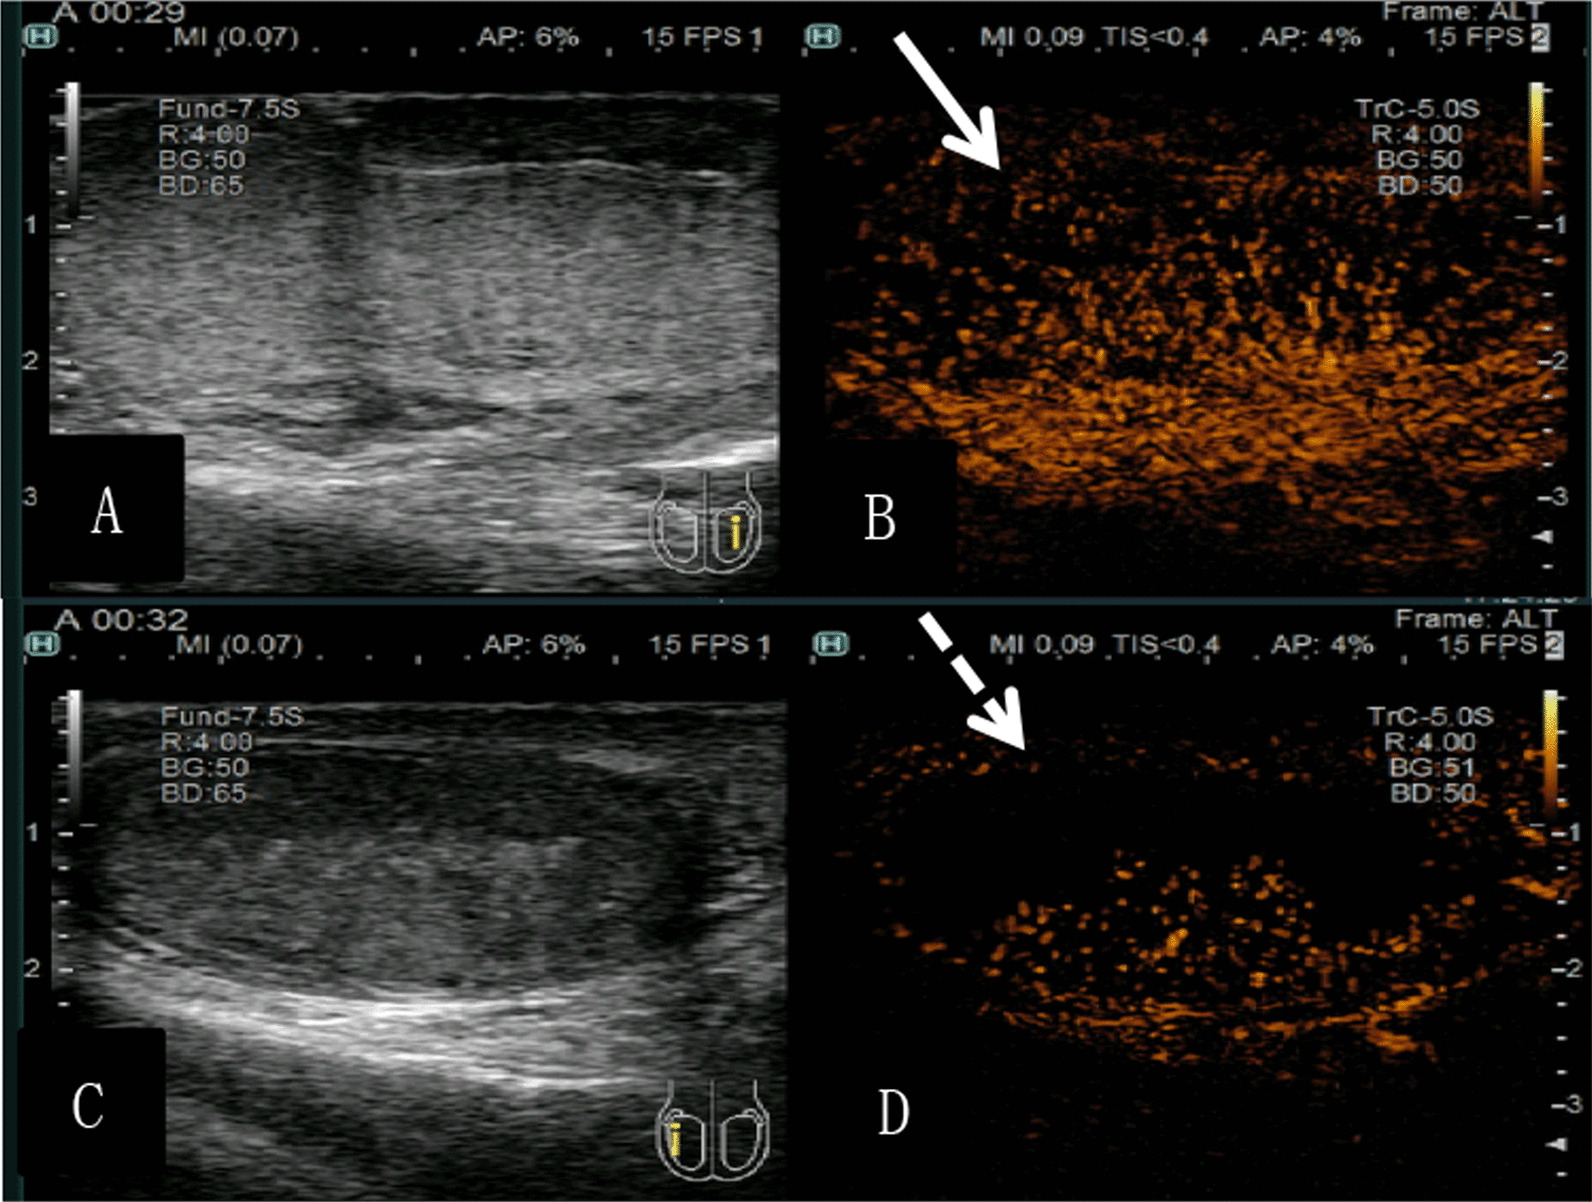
Normal testis using high-frequency ultrasound (A) and CEUS (B), testicular torsion using high-frequency ultrasound (C) and CEUS (D).
